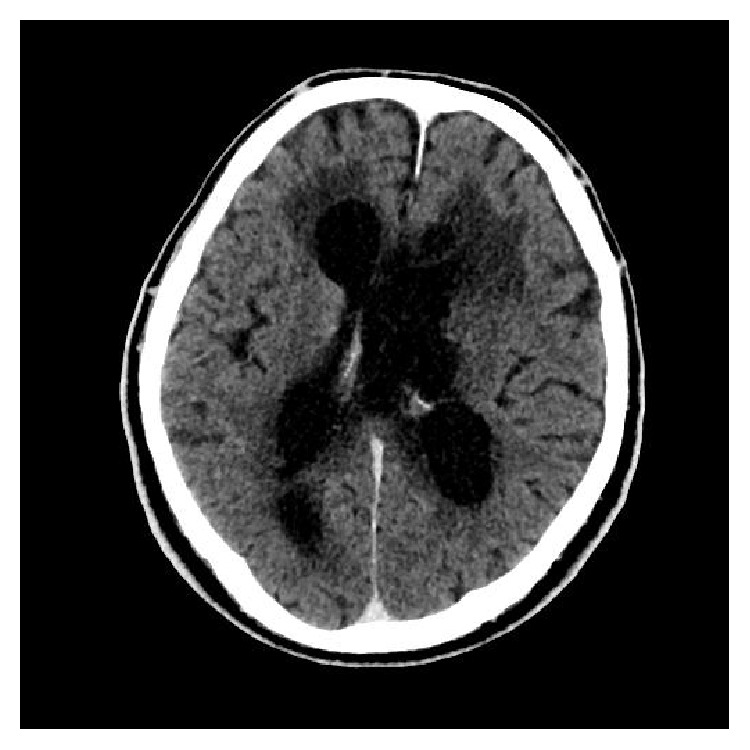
CT of the brain with intravenous contrast 6 months after admission showing progression of hydrocephalus.
